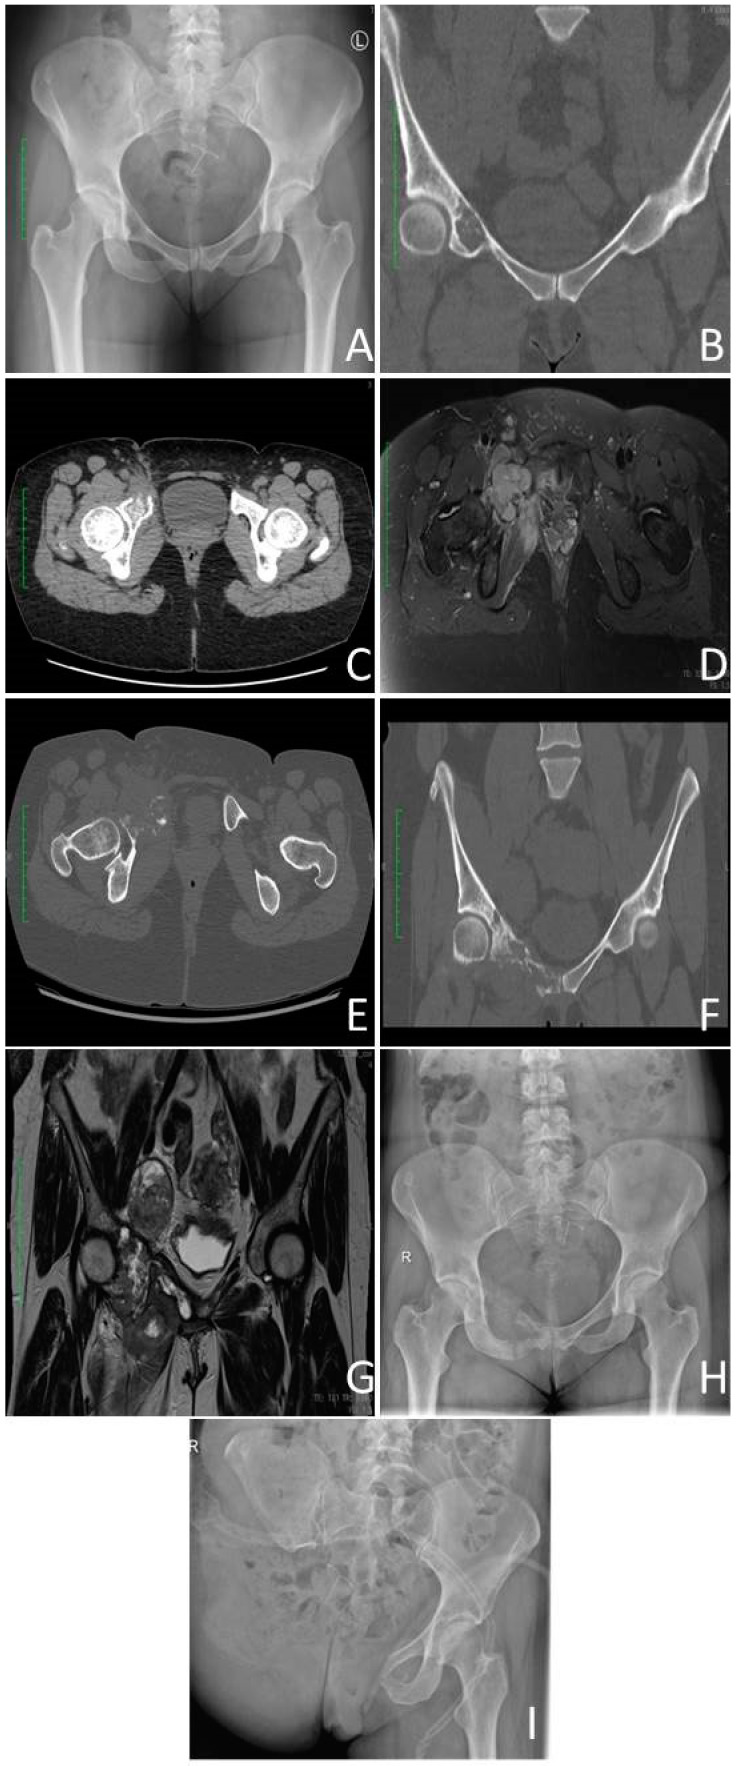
Dedifferentiated high-grade chondrosarcoma of right pubic bone. Initial presentation of the chondral lesion located in the right pubic bone, abutting acetabulum (October 2021): (A)-Anterior–posterior (AP) X-Ray view of the pelvis; (B) Coronal computed tomography (CT) view. (C)-CT Axial view—growing lesion on the follow up CT did not alert necessary attention (January 2022). (D) Contrast-enhanced magnetic resonance imaging (MRI) with fat saturation showing enlarging lesion on the follow up (August 2022). (E,F) Coronal and axial images of the progressing right pubic lesion on contrast enhanced CT scan. (G,H) Preoperative contrast-enhanced MRI coronal view scan and X-ray AP view showing lesion of the right pubic bone that progressed over 14 months (November 2022). (I). Postoperative image after right hind-quarter amputation.
